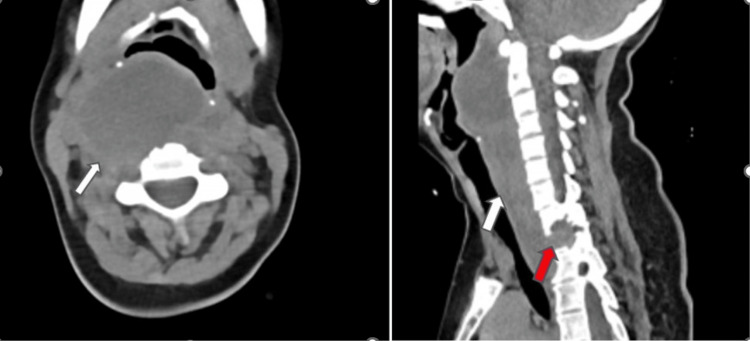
Axial and sagittal non-contrast CT scan of the neck shows a large hypodense collection (white arrow) in the retropharyngeal space extending from the skull base to the D3 vertebral level displacing the oropharynx and esophagus anteriorly. Lytic partial destruction of the lower half of the body of the D1 vertebra and complete destruction of the body of the D2 vertebra is also noted (red arrow)
